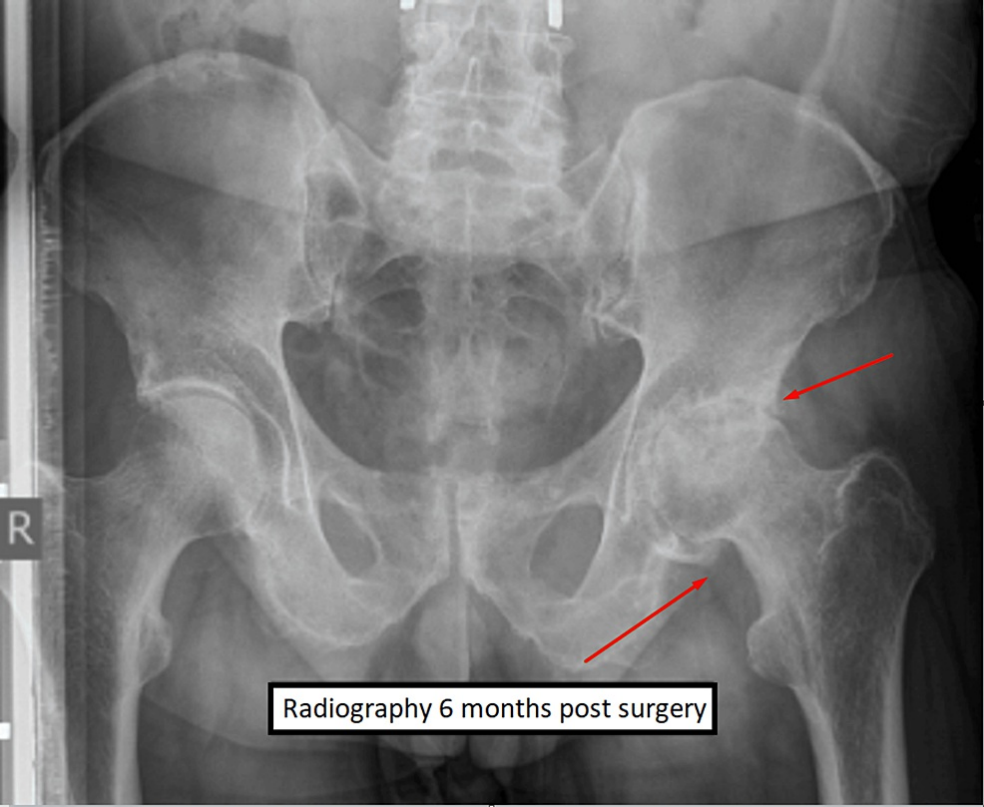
Radiography six months post-surgery Radiography showed evidence of coxarthrosis of the left hip joint
